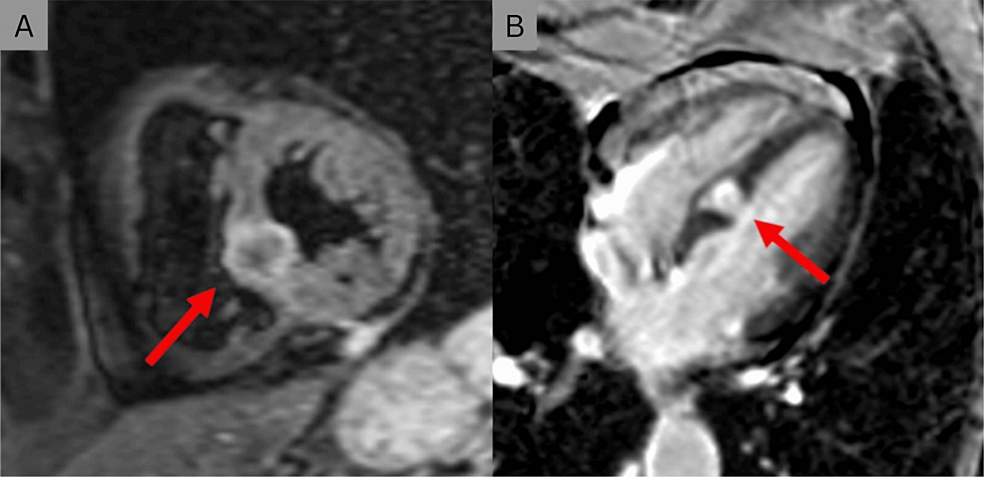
(A) Cardiac MRI scan in sagittal view may reveal a highly intense tumour within the septum between the ventricular chambers (red arrow). (B) After gadolinium injection, an axial cardiac MRI scan shows a granular improvement inside the interventricular septum (red arrow). Image Credit: Jumeau et al., 2020 [45]; Licensed under CC-BY
